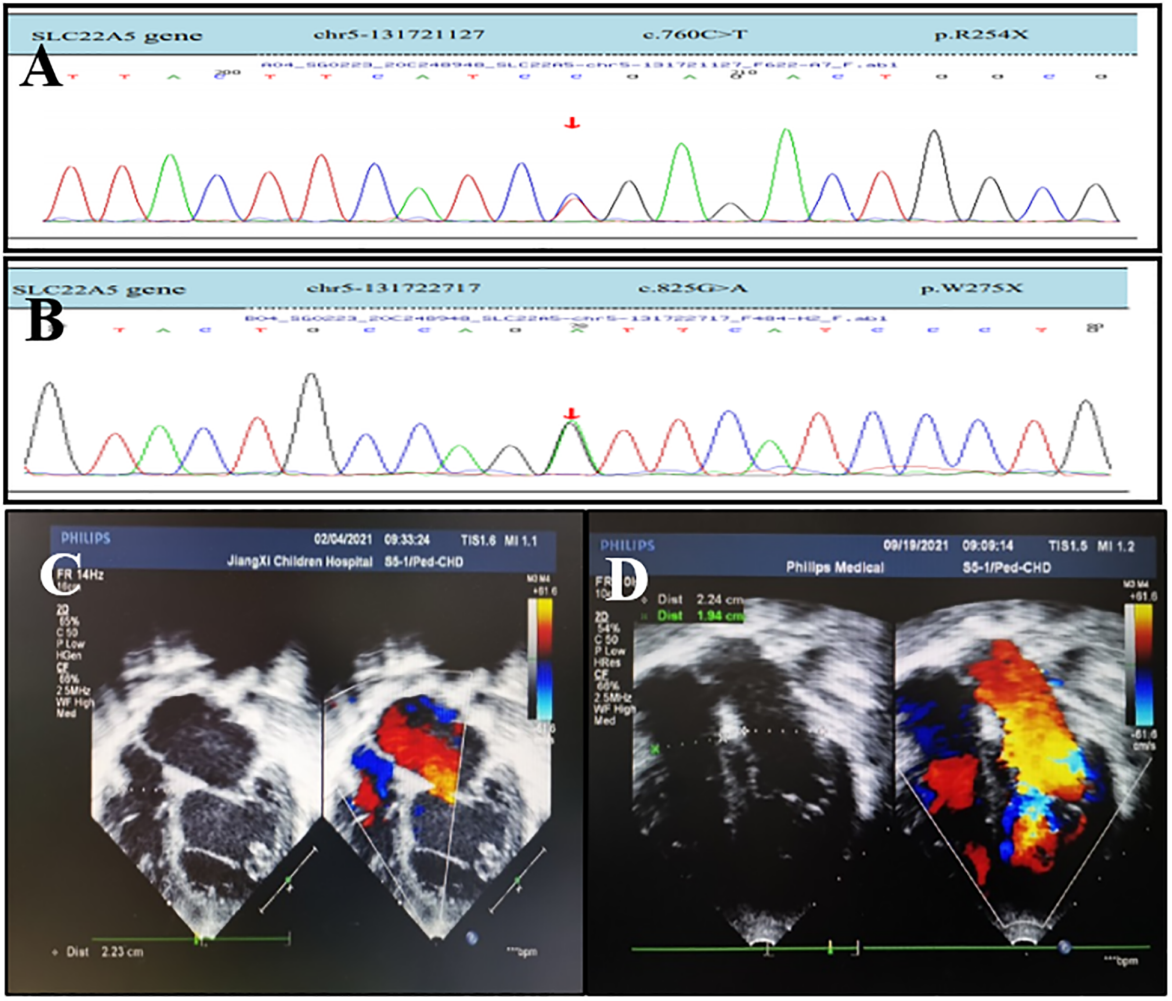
OCTN2 mutations and echocardiography of a 6-year-and 4-month-old boy (the proband's brother) with primary carnitine deficiency. (A) Mutation [c.760C > T (p.R254X)] in the *SLC22A5* gene. (B) Mutation [c.825G > A (p.w275X) ] in the *SLC22A5* gene. The arrows indicate the site of the mutation. (C) Transthoracic echocardiography (TTE) of the boy was performed in February 2021 when he was 6 years and 4 months old and did not receive treatment. (D) TTE of the boy after 6 months of treatment in September 2021. TTE parasternal four-chamber view shows diameter changes of heart chambers before and after 6 months of treatment.
